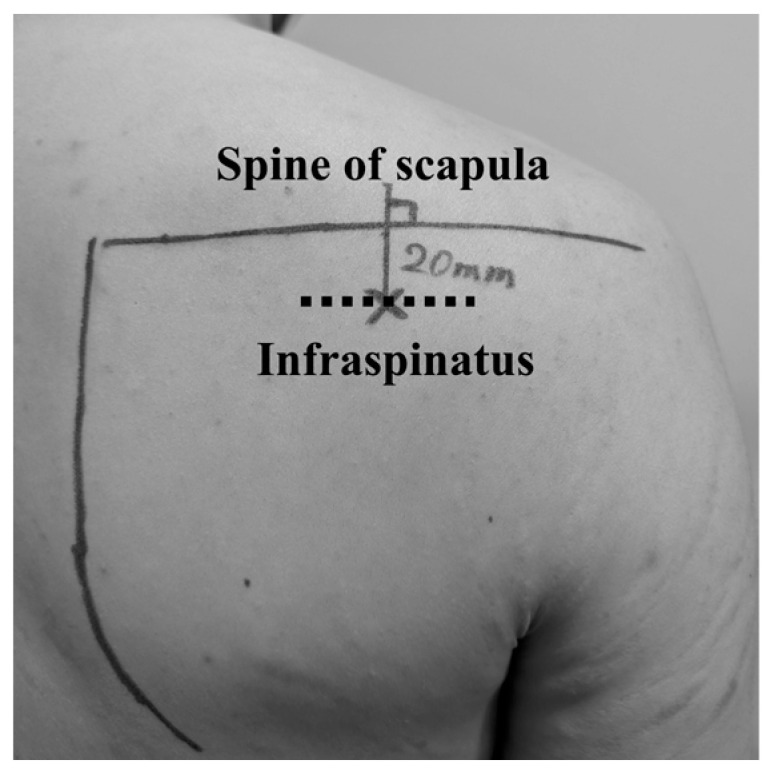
Measured points of ultrasound examinations.
